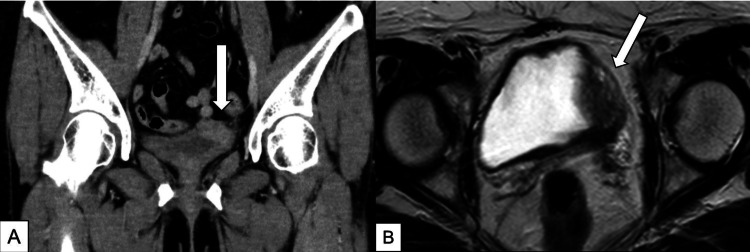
Abdominal CT (A) and pelvic MRI (B) revealed a tumor lesion with partial extravesical infiltration on the left wall of the bladder CT: computed tomography; MRI: magnetic resonance imaging
